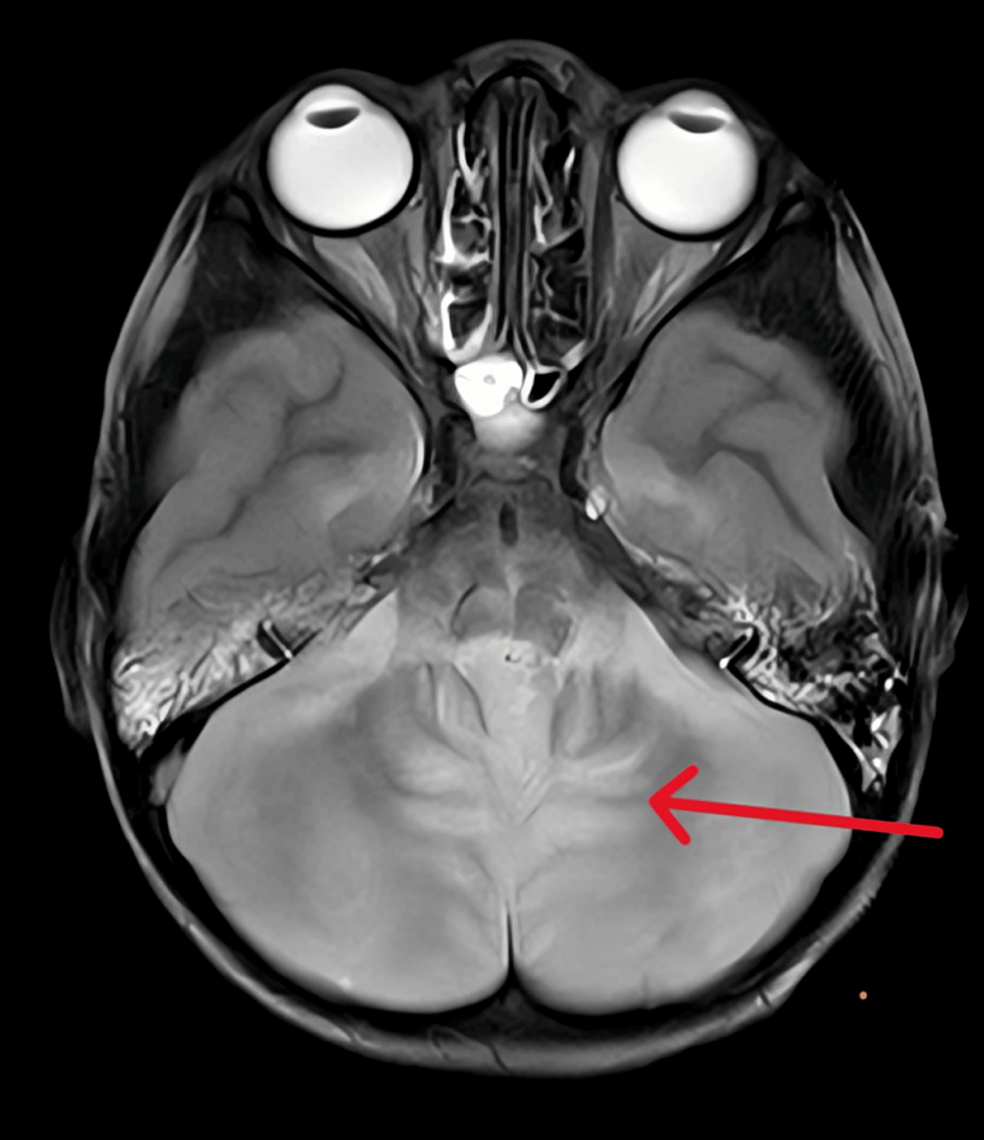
MRI (T2-weighted image/FLAIR) axial view showing mild edema with diffuse hyperintensity in the cerebellum (shown with red arrow) with associated leptomeningeal enhancement suggestive of acute cerebellitis. FLAIR: fluid-attenuated inversion recovery.
